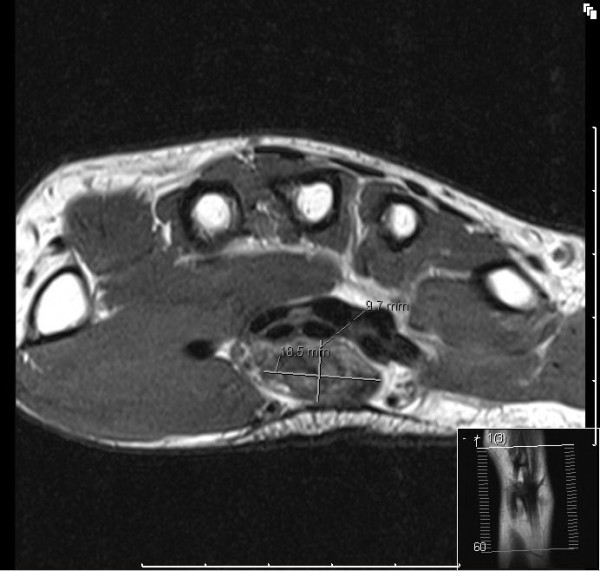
MRI scan showing the suspicious swelling (18.5 mm × 9.7 mm) within the carpal tunnel.
